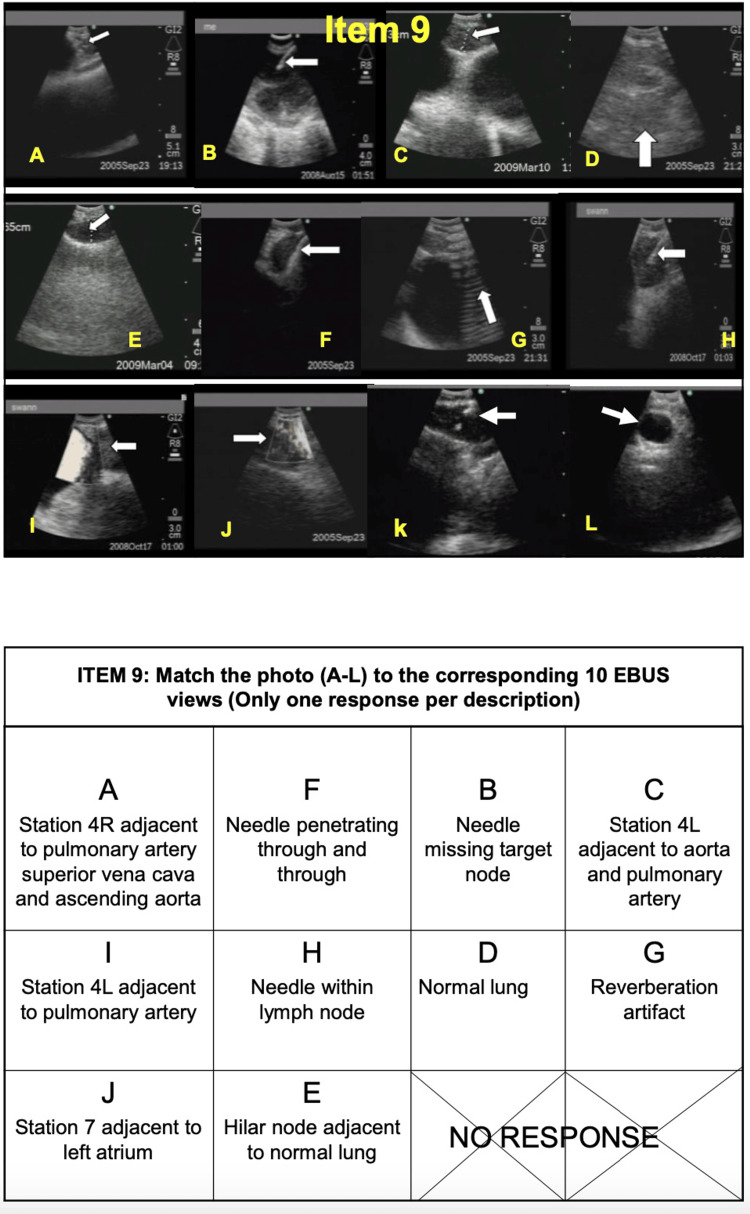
EBUS-STAT item 9 (EBUS image interpretation). EBUS-STAT: Endobronchial Ultrasound Skills and Task Assessment Tool. Obtained permission for publication and adapted from https://www.bronchoscopy.org/downloads/BEP%20EBUS%20assessment%20tools.pdf [13].
